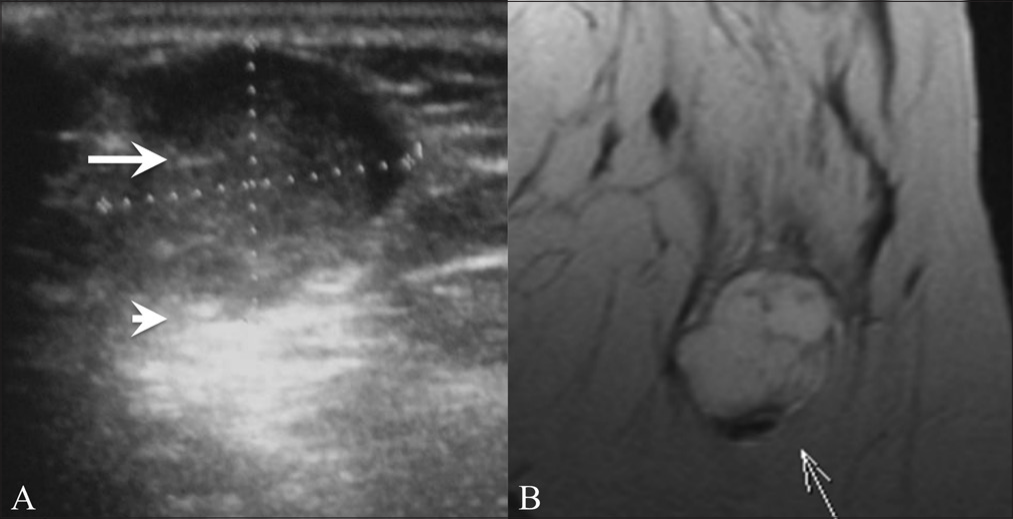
Mucinous carcinoma. It is well-circumscribed on USG(A) with echogenic internal contents (arrow) and posterior enhancement (arrowhead). (B) The mucin shows increased intensity (arrow) on a T2W MRI image
